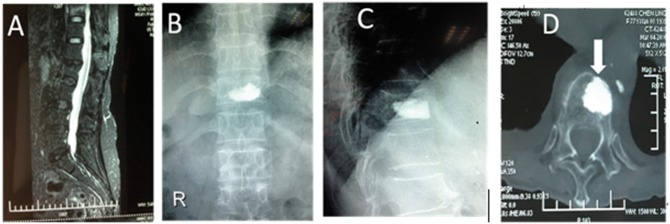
A 77-year-old patient underwent PKP for T11 osteoporotic vertebral compression fractures. (A) Preoperative MRI shows the compression fracture of the T11 vertebral body. High signal intensity is shown in the fat-suppression image. (B, C) Anteroposterior and lateral projection re-examination performed 3 days postoperatively. (D) CT scan performed 3 months postoperatively shows a clear boundary between the bone cement and bone tissues (arrow).
